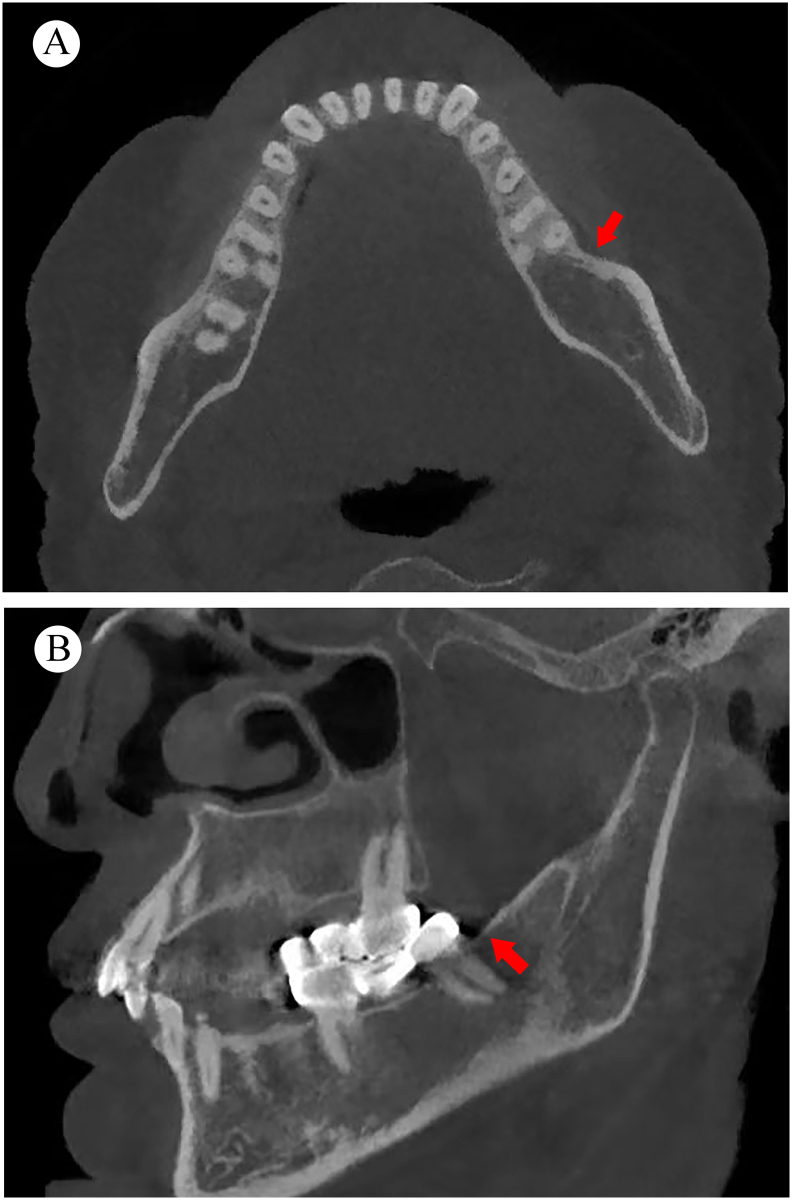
Cone beam computed tomography shows that the cortical bone of the left mandible is continuous without any obvious abnormality (red arrow). The axial plane (A) and the sagittal plane (B) images are shown here. (For interpretation of the references to color in this figure legend, the reader is referred to the web version of this article.)
